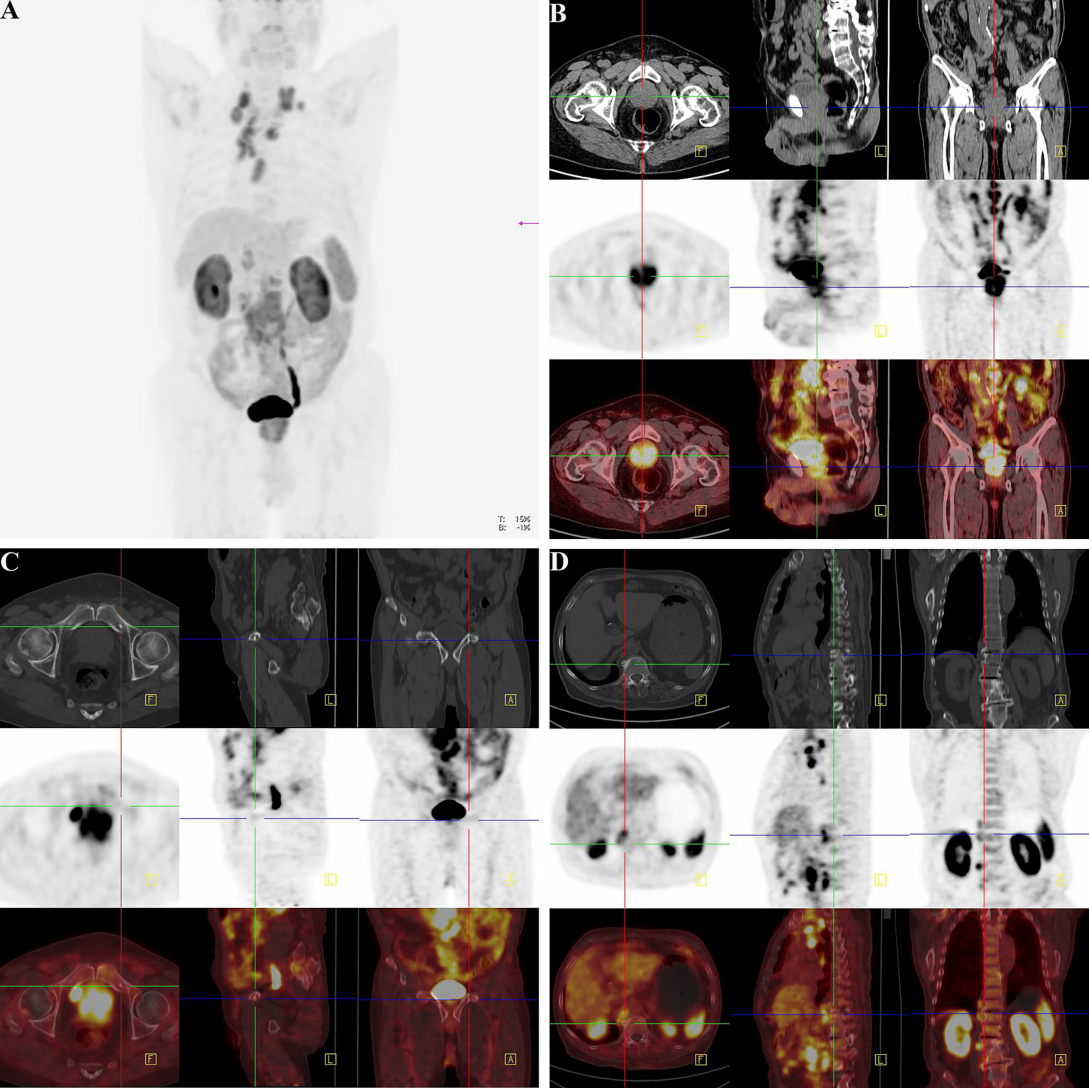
^18^F-Alfatide II as the molecule probe of integrin αvβ_3_ revealed high intake in primary and metastatic lesions through the PET-CT detection in a case of 82-year-old man. (A) Gross appearance of coronary position detected by PET-CT. The darker the color was, the higher the intake of tracer in the corresponding body part. (B) The direction cross focused on the primary lesion in prostate. The first row were CT views centered on high intake lesion of PCa from three different directions in the pelvis, which revealed the adjacent organs and structure. The second row were the PET-CT views detected by ^18^F-Alfatide II as the molecule probe of integrin αvβ_3_ centered on high intake lesion of PCa from three different directions. As the main function of bladder should be storing urine and the tracer was discharged by urine, it was rational that there was darker high-intake region in the bladder. However, the abnormal area of high-intake tracer appearing in the prostate suggested high-level affinity of probe which might be closely correlated to malignant cancer. The third row was combined with the figures of the first and second rows. The lighter the color shows the higher the intake of tracer in prostate. (C) The direction cross focused on the metastatic lesion in the middle part of the pubis from three differential directions. The first row was the view of regular CT, which revealed the bone structure and surrounding tissues. There was an unusual small spot of osteoproliferation in the direction cross. The second row demonstrated the abnormal high-intake lesion of ^18^F-Alfatide II in the left side of the middle part of the pubis, respectively, which was highly coincident with the “unusual small spot”, The third row was the combination of the first and second row, which systematically reported the accurate location of metastatic lesion in pubis and relative extent of tracer intaking. (D) The direction cross focused on the metastatic lesion on the right side of the lumbar 1 vertebra. The first row was regular CT view in the slice of abdomen. Compared with the adjacent vertebrae, the direction cross revealed high density in bone cortex, which was consistent with the characteristics of osteoblastic metastasis in PCa. In the second row, from the sight of ^18^F-Alfatide II, except for the rich blood supply organs like kidneys, spleen, and liver, the strange high intake of probe in the vertebra presented metastatic lesion. In the third row, the emerge of both first and second rows in the same slice then provided the reliable evidence of metastatic lesion form the aspects of position and signal strength.
